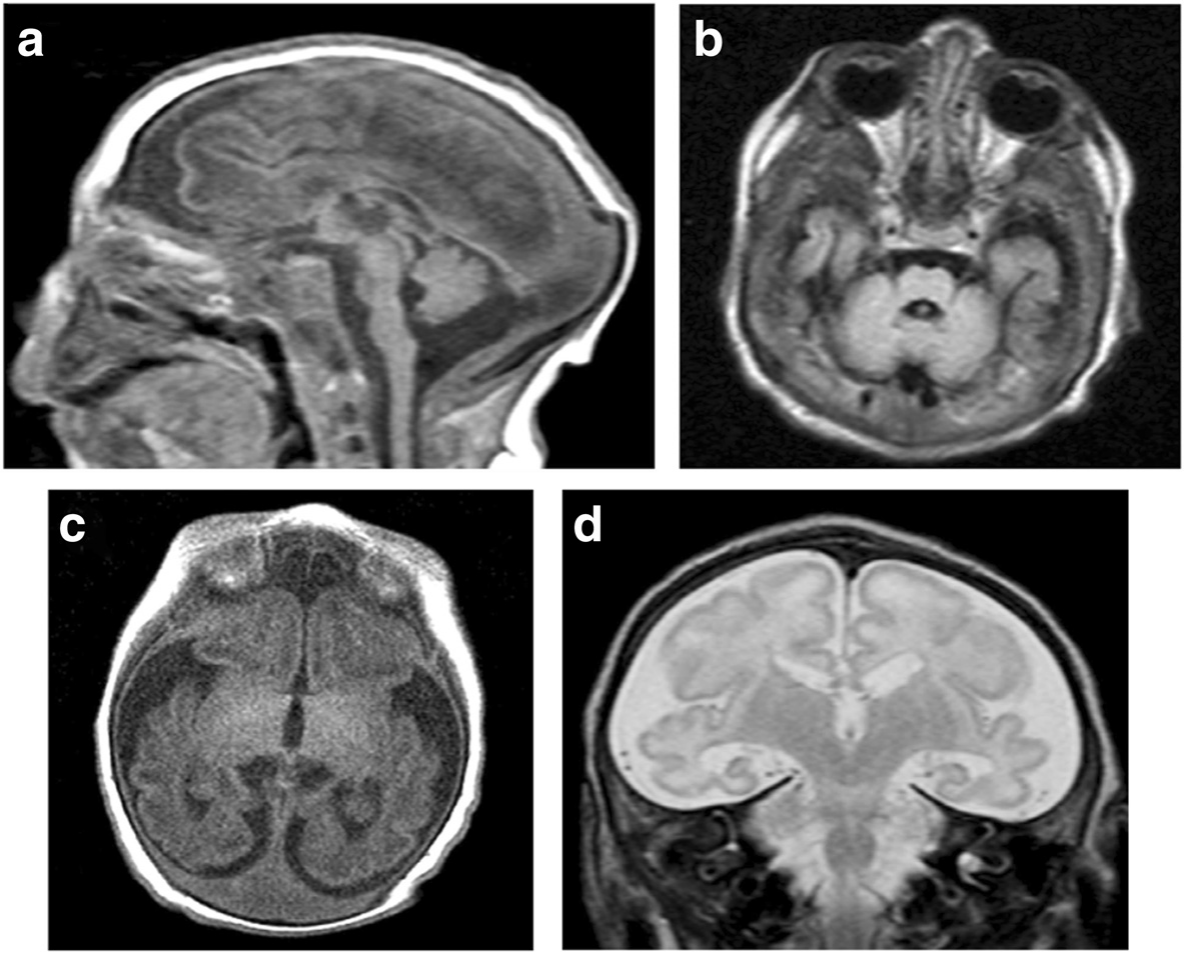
MRI brain at the age of 3 weeks. a Sagittal T1-weighted image showing severe microcephaly with overlapped lambdoid sutures, brain underdevelopment evident by simplified gyral pattern, small cerebellum, and small pons. b Axial FLAIR image revealing small pons and cerebellum. c Axial T1-weighted image demonstrating delayed myelination evident by absent myelination of the posterior limb of internal capsule, bilaterally. d Coronal T2-weighted image showing simplified gyral pattern (more in the frontal lobes) and large extra-axial CSF spaces due to brain underdevelopment
